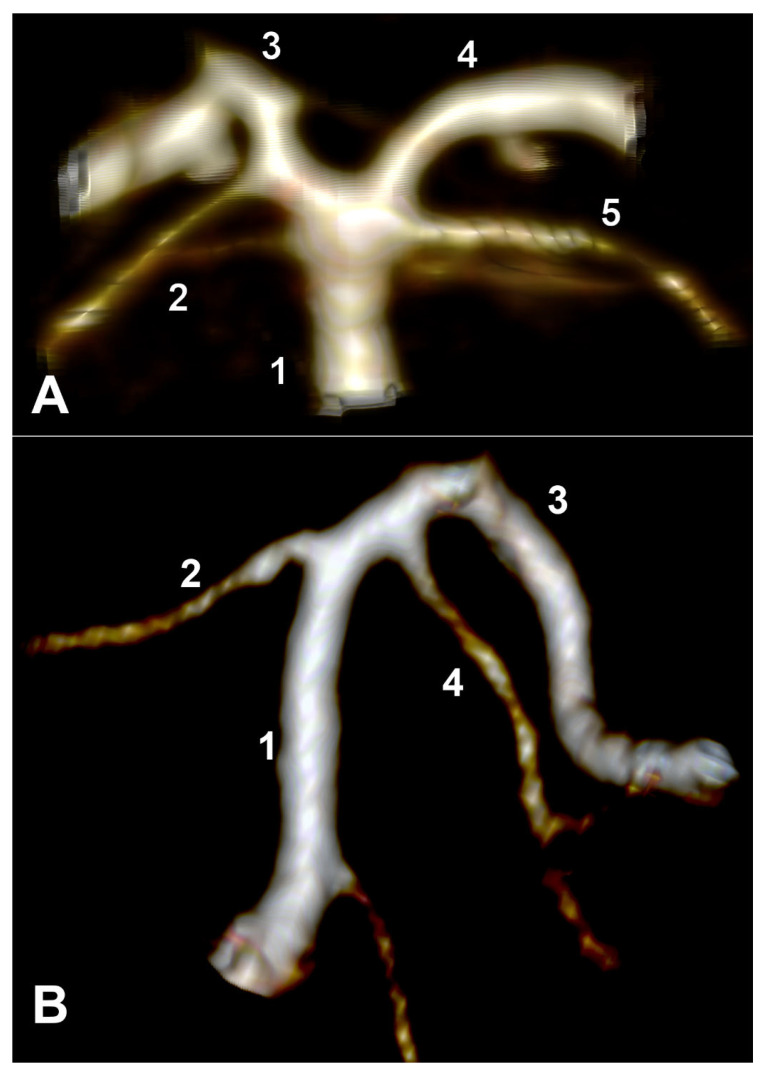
Type G of the bilateral combination of superior cerebellar artery origins. Posterior views of basilar arteries. CT angiograms with three-dimensional volume renderings. (A) Bilateral normal PCAs. 1. BA; 2. left SCA; 3. left PCA; 4. right PCA; 5. right SCA. (B) Aplastic P1 segment of the left PCA. 1. BA; 2. left SCA; 3. right PCA; 4. right SCA.
